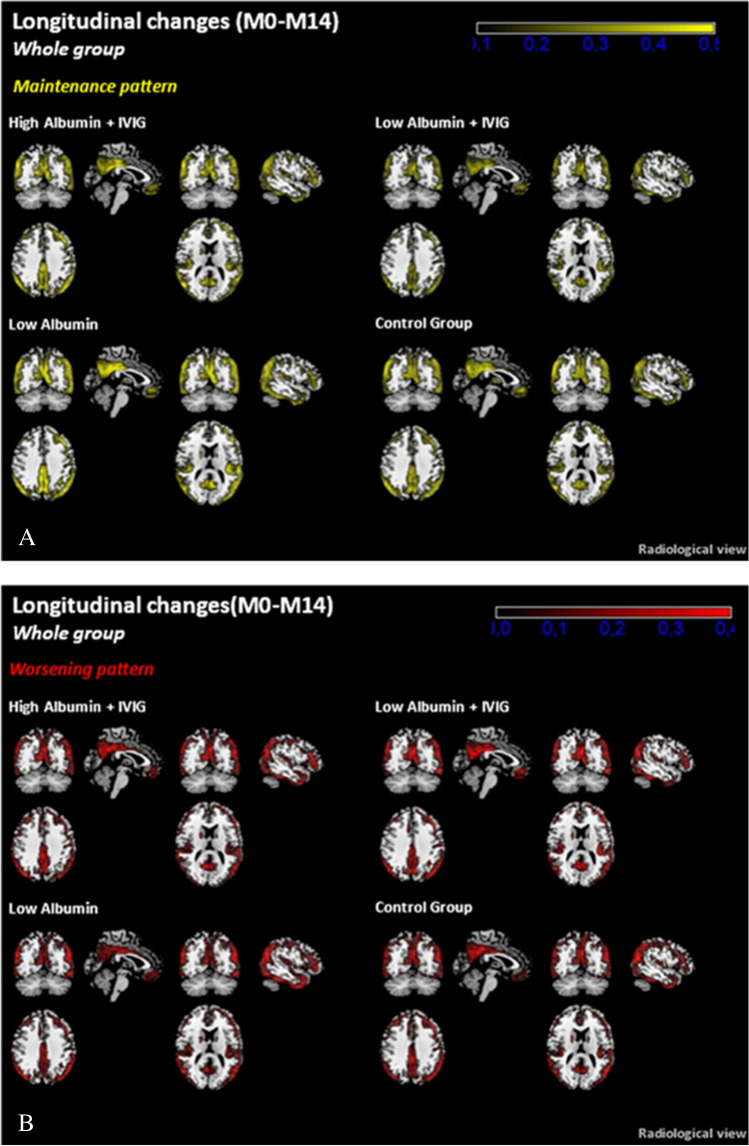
Triangulation view of the metabolic maintenance pattern (A: upper panel) and worsening pattern (B: lower panel) (parametric ^18^FDG-PET analysis) over the complete study period (baseline [M0] to month 14 [M14]) in Alzheimer’s disease patients treated with plasma exchange with low- or high-dose albumin with or without intravenous immunoglobulin (IVIG). All patients
